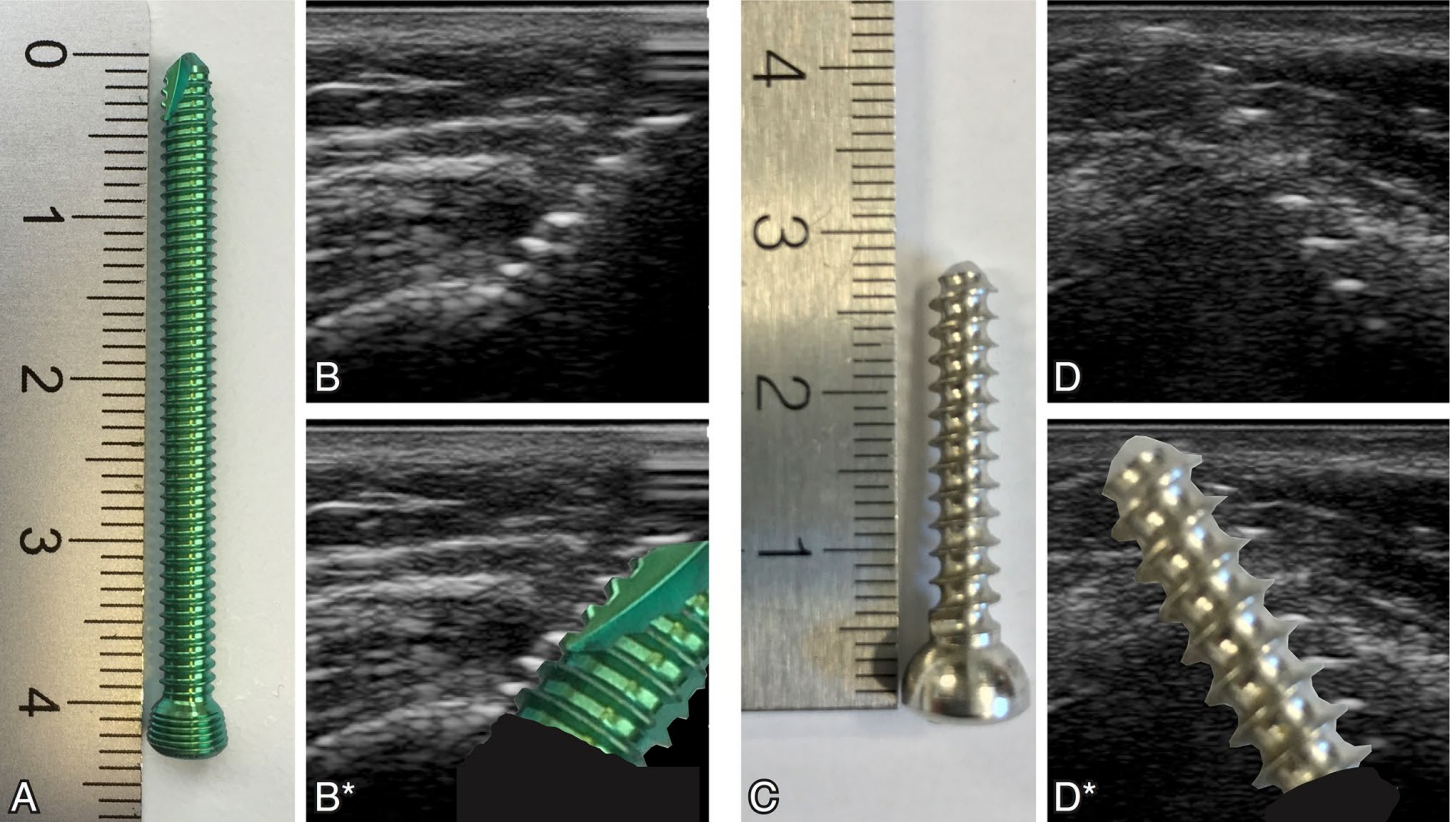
Types of screws used in the study. A: Self-tapping (thread cutting) screw of 3.5 mm diameter. B: Ultrasound-image of the self-tapping screw, B* same image with the photo of the screw tip overlapped. C: Regular (non-cutting) screw of 4.5 mm diameter. D: Ultrasound-image of the regular screw, D* same image with the photo of the screw tip overlapped. The reflections on the screw threads are clearly visible in the ultrasound image (B and D) as regularly arranged bright hyperechogenic lines
